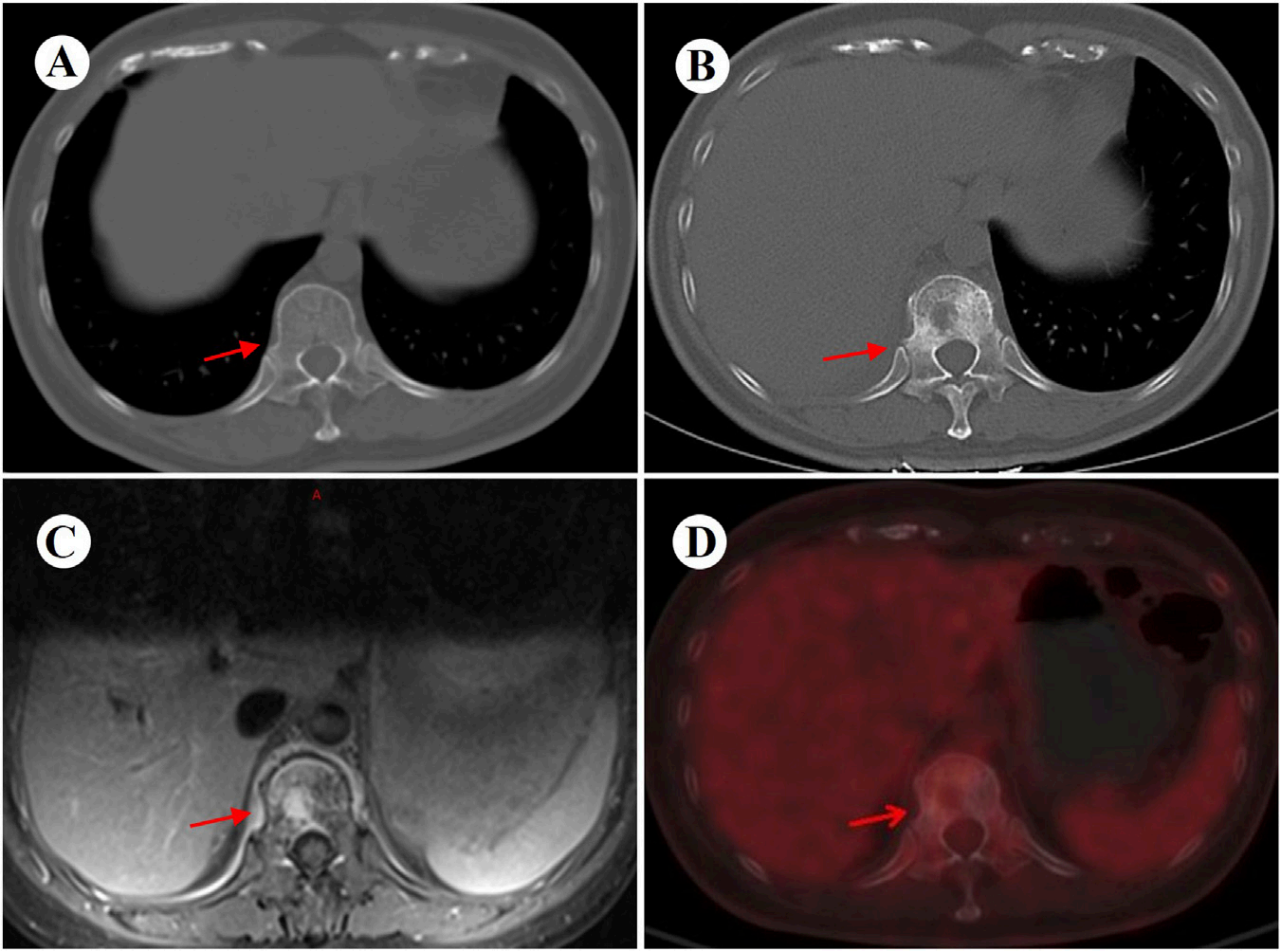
Images before and 3 months after surgery. (A) Preoperative chest CT (bone window) performed on 13 March 2024 shows an intact T12 vertebral body and attachments. (B) Follow-up CT (bone window) at 3 months postoperatively reveals new osteolytic destruction of the T12 vertebral body and attachments. (C,D) Subsequent MRI and PET-CT further confirm metastatic involvement of the T12 vertebra, with concordant imaging findings. CT, computed tomography; PET, positron emission tomography; MRI, magnetic resonance imaging.
